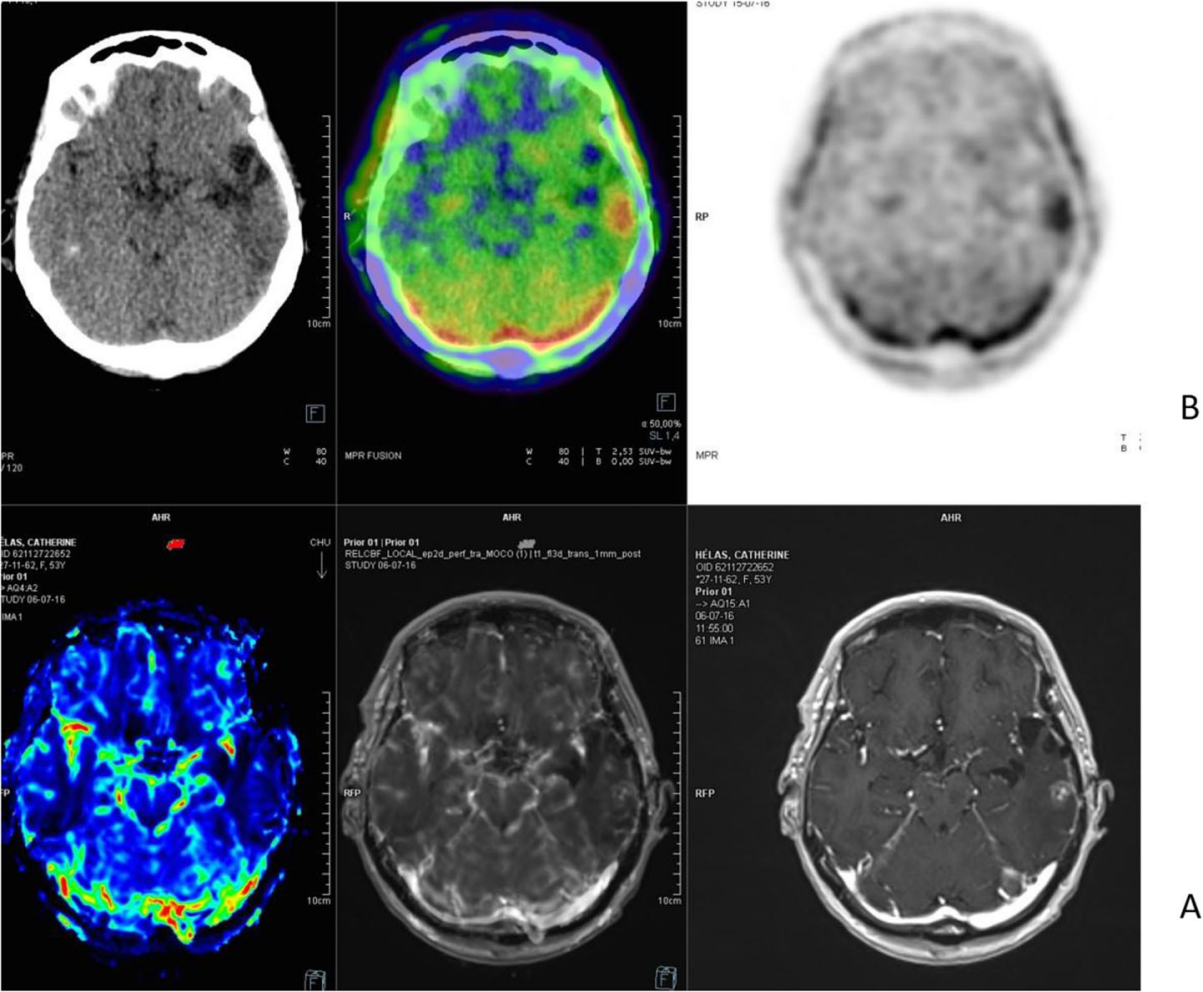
^18^F-FET, suspicion of recurrence. *Clinical history*: 55 y.o. woman, with grade II–III oligo-astrocytoma treated by surgery and adjuvant radiation therapy 13 months prior. MRI shows a focal area of Gd enhancement but no corresponding perfusion anomaly, consistent with radiation necrosis (a). *PET/CT findings*: highly increased FET uptake in the lesion, consistent with persistent/recurrent tumour on PET and fused images (b). Surgery revealed grade II oligo-astrocytoma
